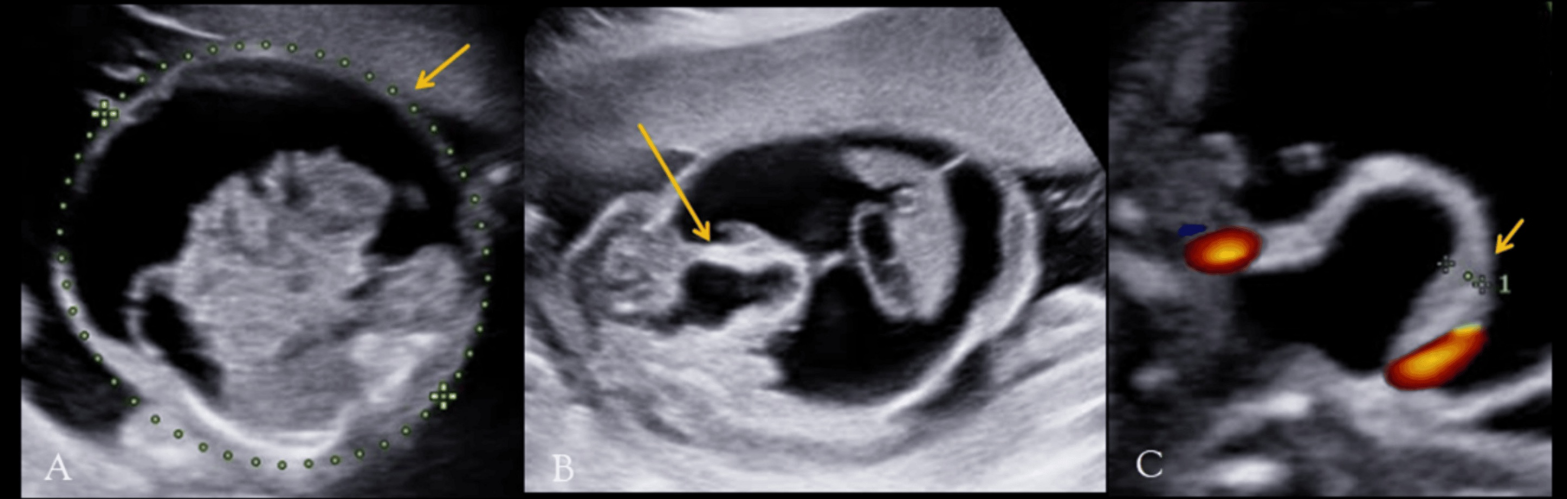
Antenatal ultrasound of the 20-week-old fetus shows increased abdominal circumference measuring 189 mm (dotted line, A), with anechoic fluid collection within the peritoneal cavity (arrow, A). The "keyhole" sign of posterior urethral valve is seen (arrow, B), and the thickened urinary bladder wall is seen (arrow, C), measuring >3.5 mm.
